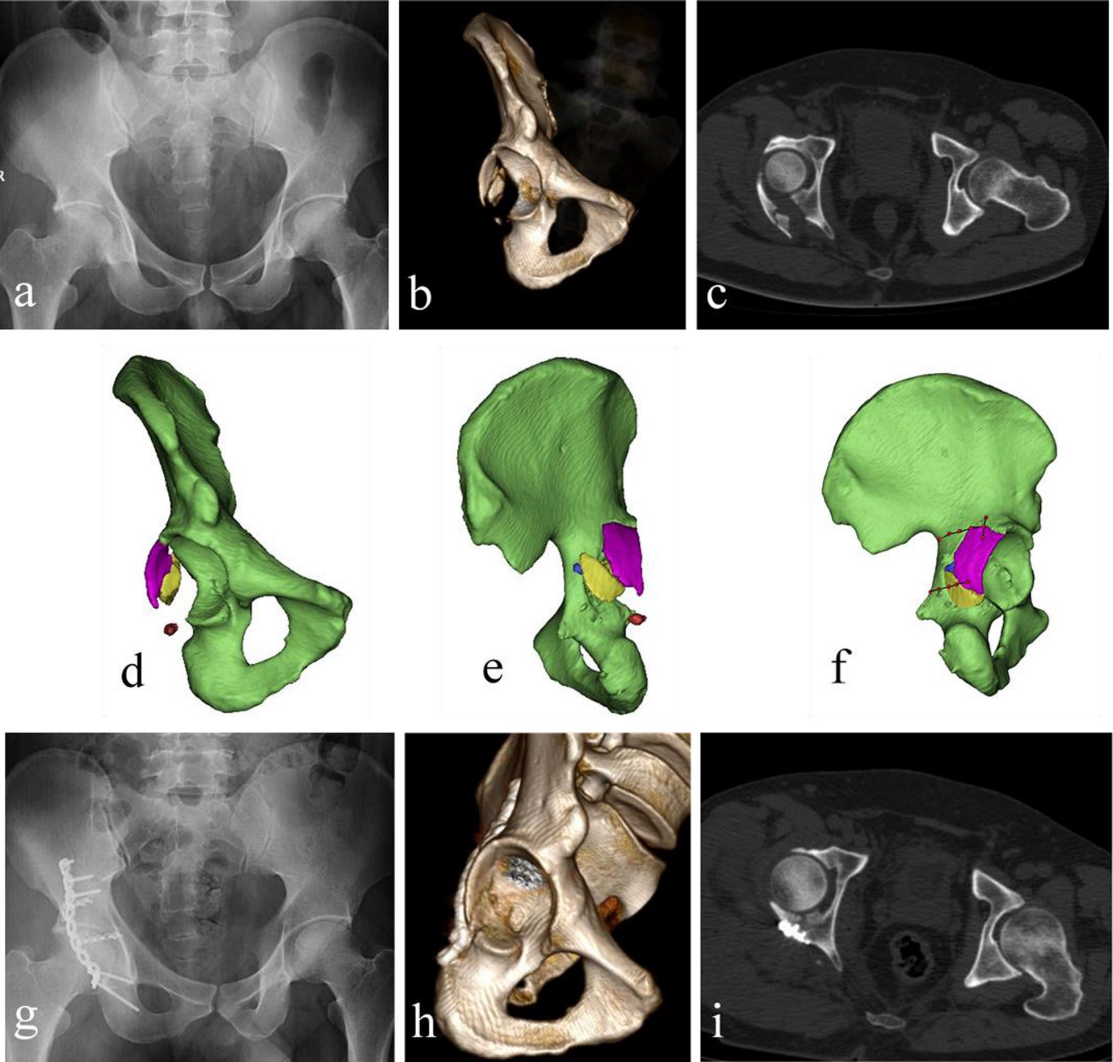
A typical case: A 40-year-old man presented with comminuted posterior wall acetabular fractures of the right acetabulum following a traffic accident. The computerized virtual preoperative planning procedures were applied for the treatment. Preoperative AP view (a), 3D-CT (b), and cross-sectional CT image (c) show comminuted posterior wall acetabular fractures with significant displacement. A 3D virtual model of right acetabulum with separate fragments was reconstructed (d, e). Preoperative planning of internal fixation methods was achieved on the post-reduction model (f). Postoperative AP view (g), 3D-CT (h), and cross-sectional CT image (i) show an anatomical reduction according to Matta grading score
